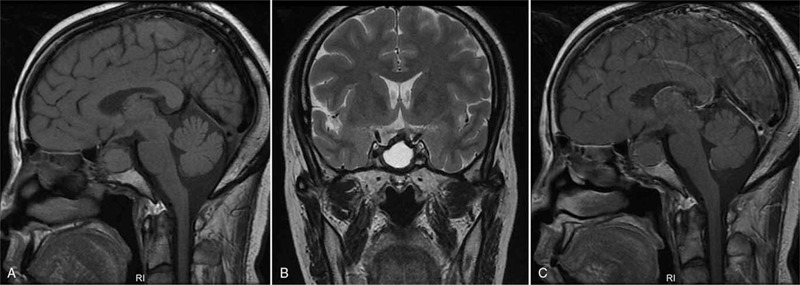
Postoperative MRI of 3 months after operation revealed complete lesion resection without residual lesion tissue (A–C). A residual cavity was formed due to postoperative fluid accumulation in the sellar region. This cavity showed hypointense on T1WI (A), hyperintense on T2WI (B), and no enhancement following the intravenous administration of gadolinium (C). MRI = magnetic resonance imaging, T1WI = T1-weighted images, T2WI = T2-weighted images.
